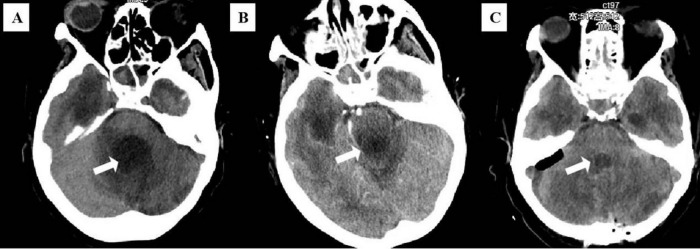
(A) Brain computed tomography (CT) images at the initial diagnosis. (B) Brain CT before the second cranial surgery on 4th day. (C) Brain CT after the second cranial surgery on 9th day. White arrows indicate the lesion in the fourth ventricle.
